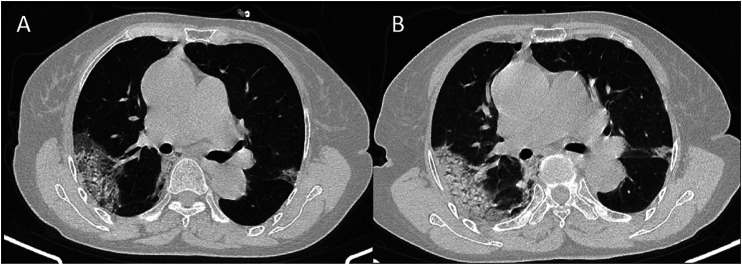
CT signs of a 74-year-old female showed subpleural distributed ground-glass opacity in 13 days (A) and 16 days (B) from onset.
